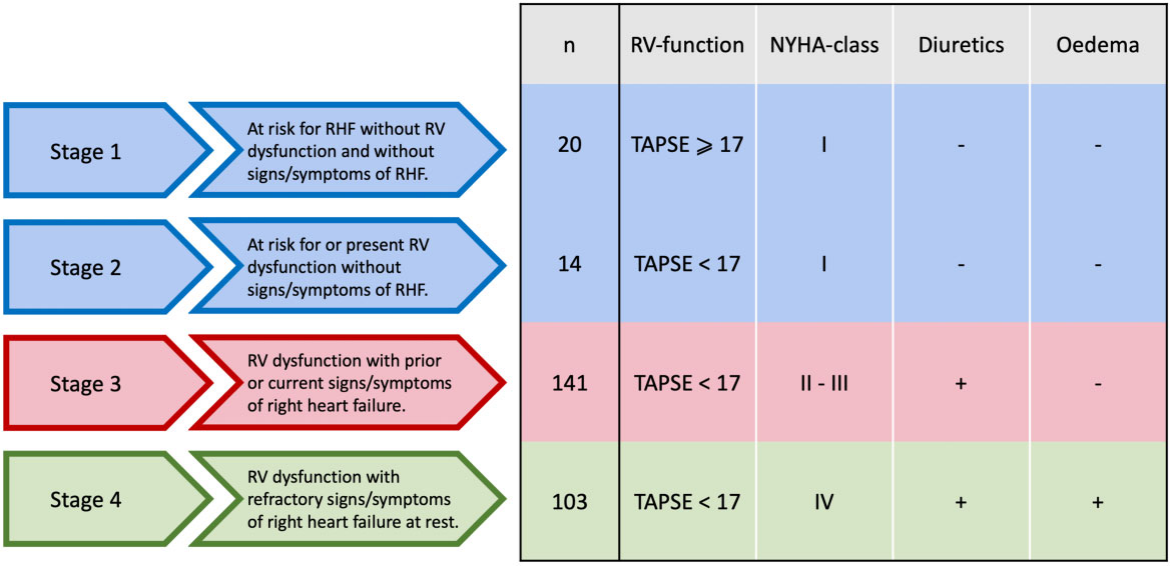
Stages of right heart failure defined by clinical and echocardiographic variables. NYHA functional class: New York Heart Association functional classification; RV: right ventricle; TAPSE: tricuspid annular plane systolic excursion.
